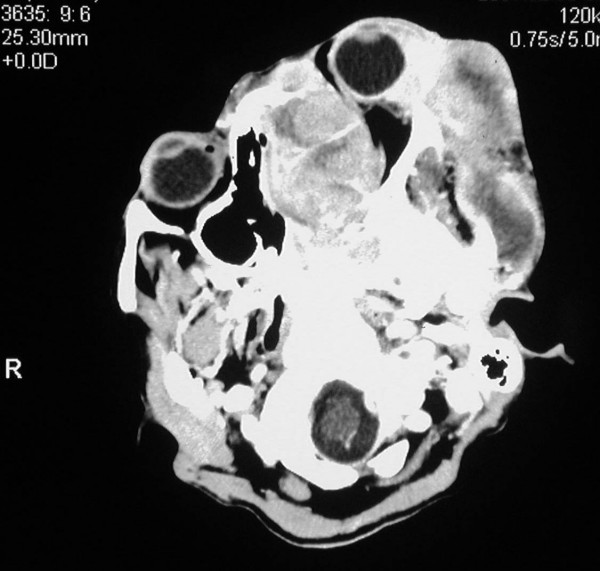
Axial CT cut demonstrating invasion of the tumour into the left orbit, causing proptosis; and the extension of the tumour to the left ethmoidal sinus and the temporal and infra-temporal fossae.
